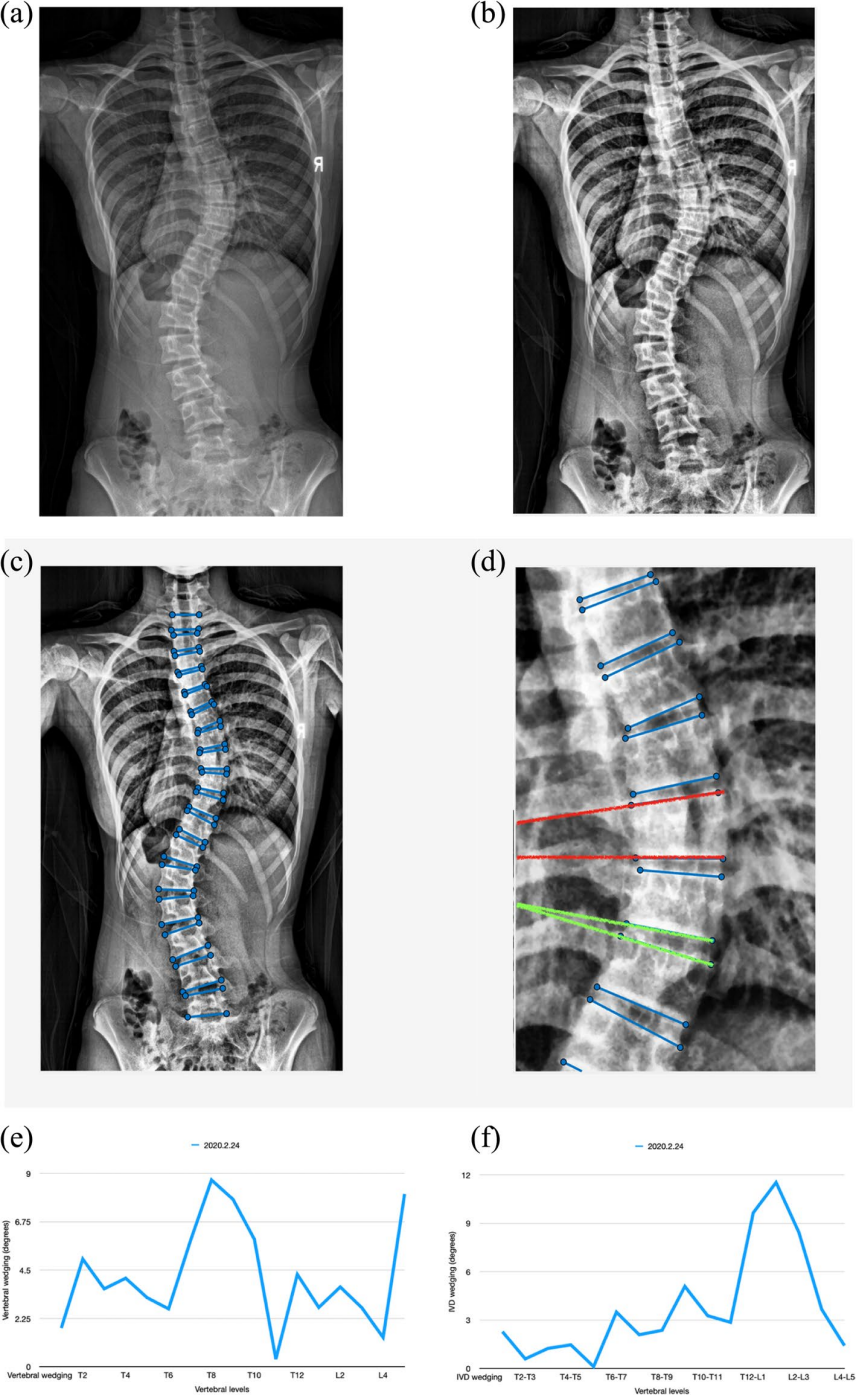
Steps in the measurement process. a Original X-ray. b After the X-ray was imported into MATLAB, a filter was used to enhance image contrast. c Lines across the superior and inferior endplates of each vertebra were drawn. d The lines passing through the superior and inferior endplate of the vertebra (red line) were used to calculate vertebral wedging while lines passing through the inferior endplate of the cranial vertebra and superior endplate of the caudal vertebra (green line) were used to calculate IVD wedging. e Vertebral and f IVD wedging were presented in a line graph to observe the change of wedging along the spine
